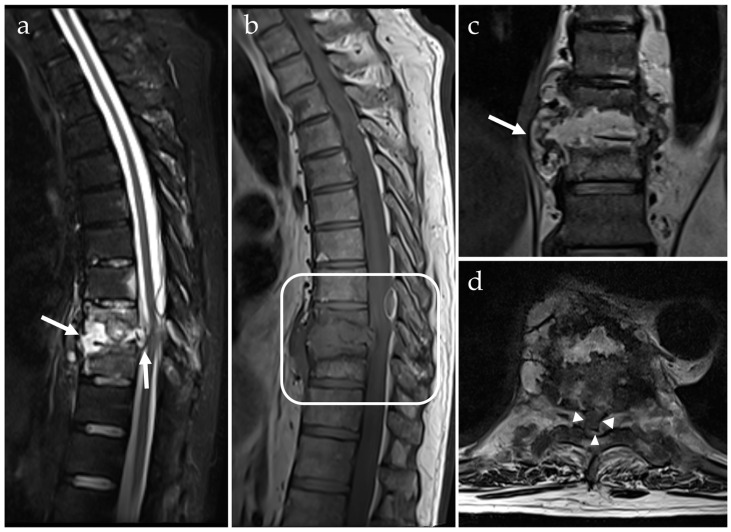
MR examination (sagittal STIR—(a); sagittal T1-weighted image—(b); coronal T2-weighted image—(c); axial T2-weighted image—(d)), depicting spondylodiscitis at the level of T9–T10: osteolysis and destruction of the intervertebral disc, with perivertebral and epidural abscesses (high signal intensity on T2/STIR and low signal intensity on T1—arrows), compressing the spinal cord (arrowheads). White case indicates the level affected by spondylodiscitis.
